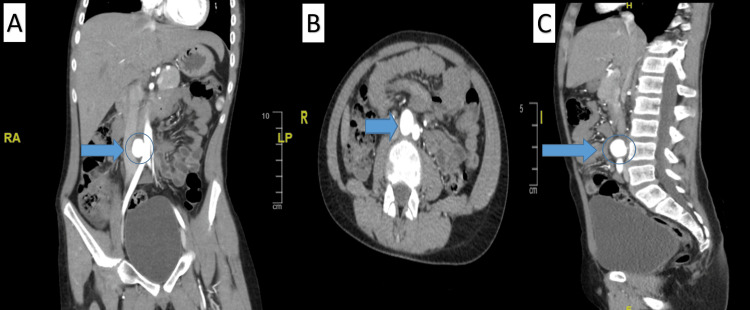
Abdominopelvic Computed Tomography Angiogram A: Coronal view (There are no signs of perianeurysmal inflammatory changes or aneurysmal leak) (blue arrow); B: Axial view (There are no signs of perianeurysmal inflammatory changes or aneurysmal leak) (blue arrow); C: Sagittal view (There are no signs of perianeurysmal inflammatory changes or aneurysmal leak) (blue arrow).
